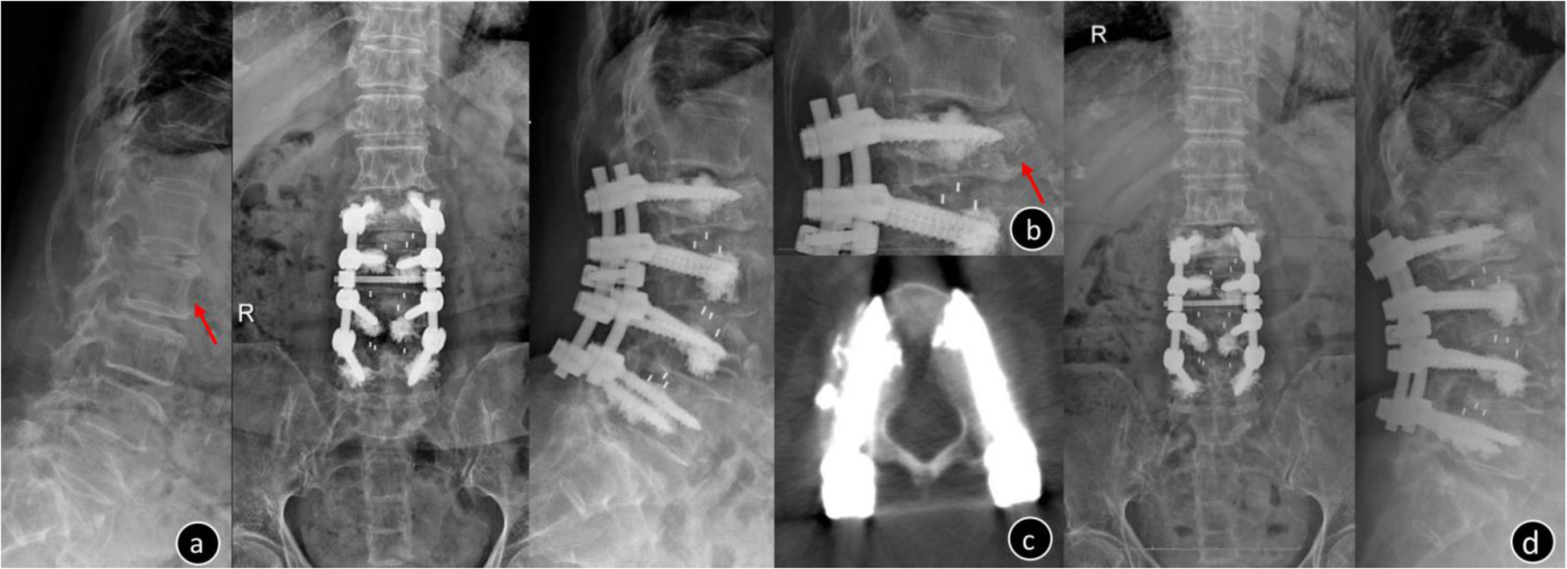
Female, 73-year-old, T = − 3.5 SD, the patient underwent solid pedicle screw with a pre-augmented trajectory a. The preoperative x-ray image showed lumbar vertebral degeneration and no fracture was found in the L2 vertebral; b. Postoperative x-ray: L2 vertebral fracture; c: when a screw was implanted late and the cement becomes solidified, the screw may deviate augmented trajectory, and the following rupture of the cortex bone may result in vertebral fracture; d. Three years after the operation, the x-ray showed that L2 vertebral body collapsed, the fracture healed and the L2 pedicle screw has not loosened
